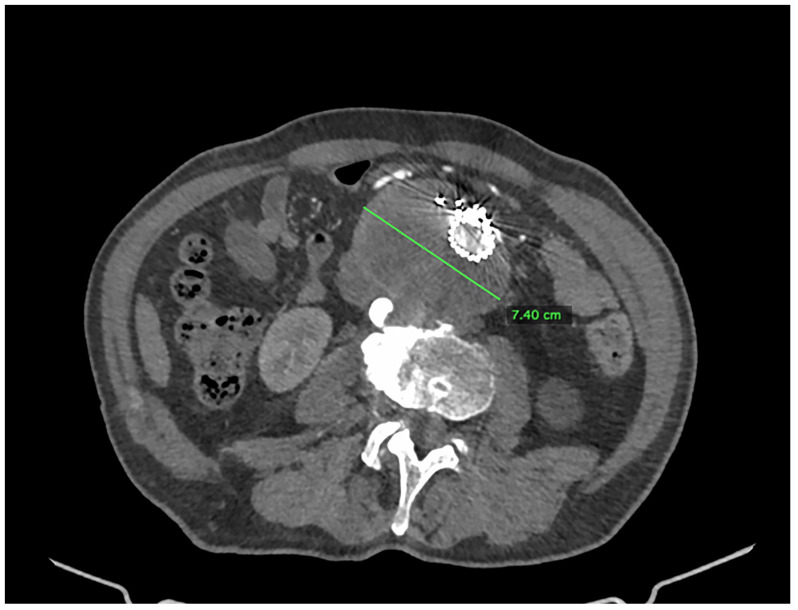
Thoracoabdominal aortic aneurysm with a visible T-Branch stent graft inside. The aneurysm diameter is marked with a green line. Pre-interventional Angio-CT scan performed at the time of the patient’s admission. Axial projection, arterial phase.
